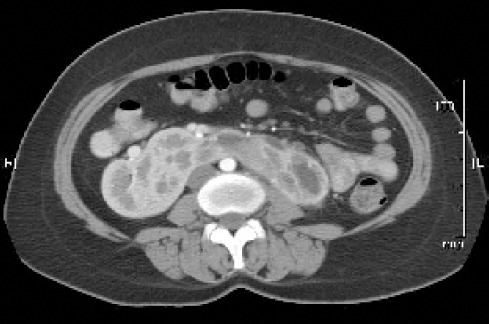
CT images showing lower moieties of both kidneys and the adjoining isthmus.
